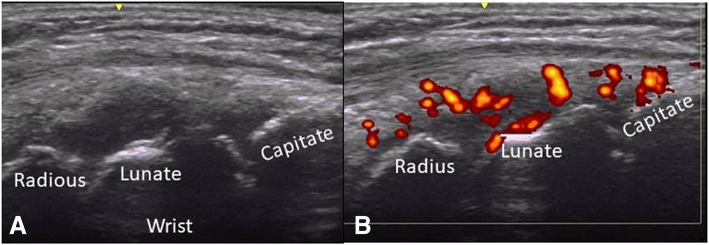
a Synovitis (grayscale) grade 2 in wrist. b Power Doppler grade 2. Both in longitudinal view
